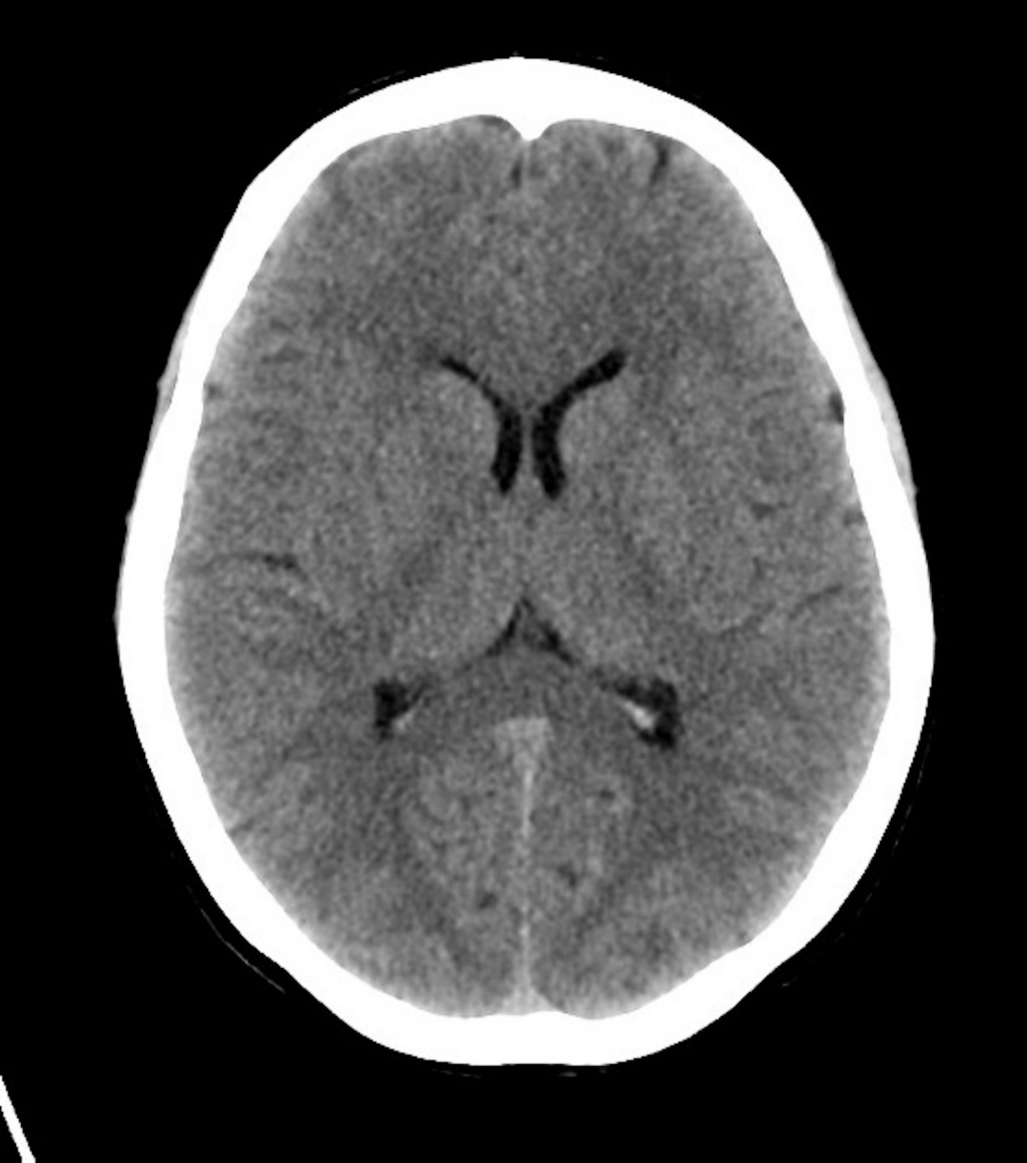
Sample normal computed tomography for a patient presenting with altered mental status secondary to metabolic alkalosis
